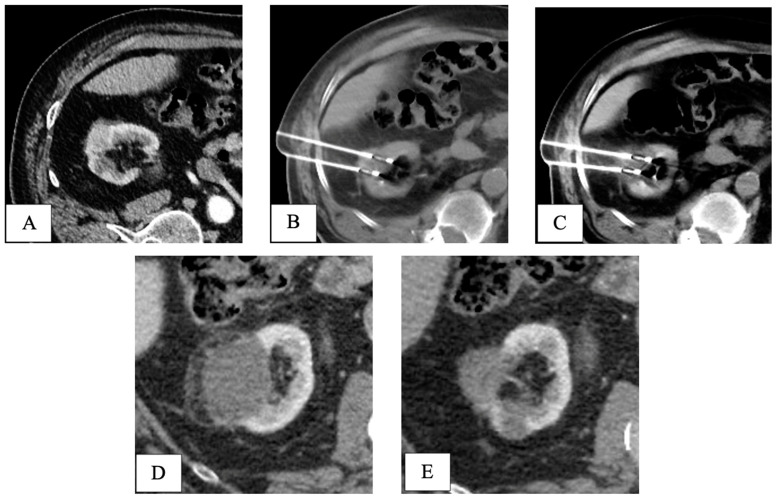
CT-guided cryoablation of de novo kidney tumor in a patient who underwent liver transplant 5 years before for HCV-related HCC. Legend: (A) preliminary contrast-enhanced CT scan confirmed the presence of a T1a DKT sizing 2cm in the right kidney; (B) under CT guidance, 2 cryoprobes were placed within the renal lesion; (C) control CT scan showed the presence of a hypodense ice-ball, including all the volume of the nodule to be treated. (D) One-month follow-up CT scan detected complete response, with a hypodense cryo-induced ablation area; (E) 3-year follow-up CT scan demonstrated prolonged response, with ablation area decreasing in size. DKT, de novo kidney tumor.
